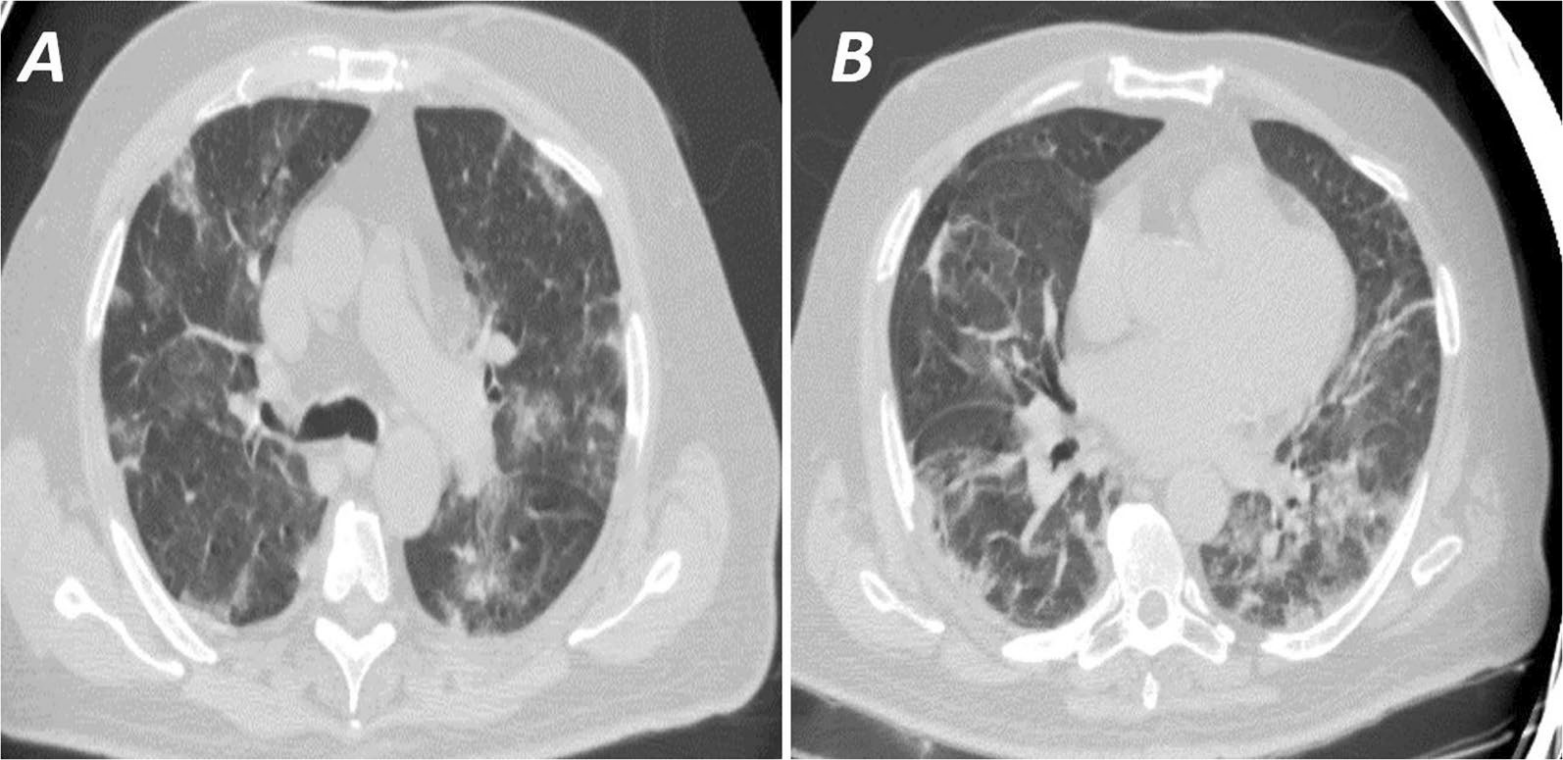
A and B High-resolution CT chest (axial cuts) for a 74-year-old diabetic hypertensive male patient presented with fever, cough, and dyspnea. CT was interpreted as typical (CO-RADS 5) by all readers. RT-PCR for COVID-19 was negative twice. The patient recovered from antibiotic treatment and was discharged a few days later
